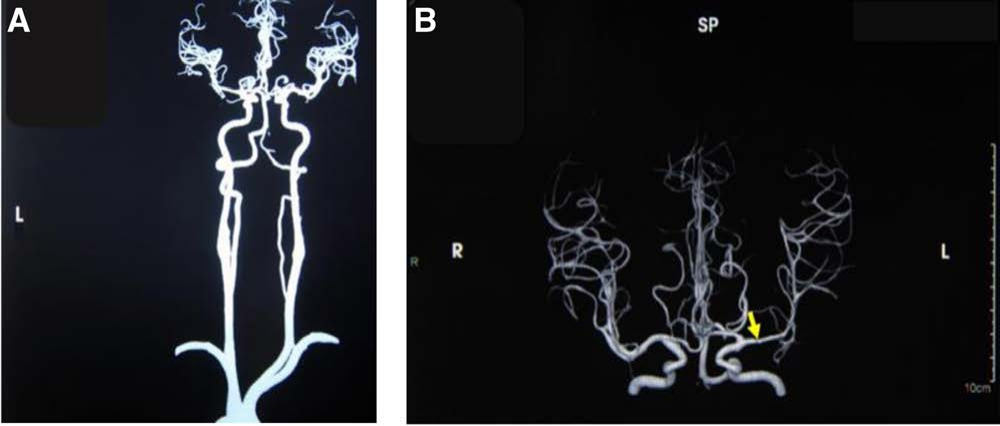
CTA showed that the right vertebral artery was fine throughout (A), and mild-moderate stenosis of the left MCA-M1 segment (B).
